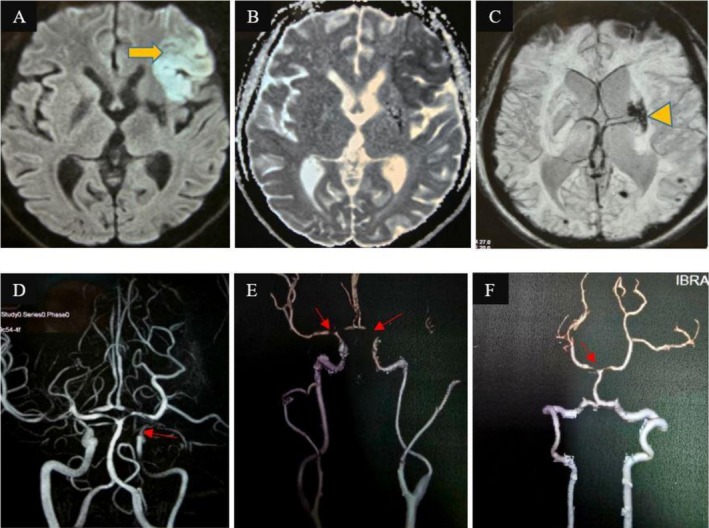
Follow up MRI of Brain, after 3 months demonstrated an area of restricted diffusion in left frontal lobe (A and B, marked by yellow arrow). Additionally, haemorrhagic transformation noted in left capsule‐ganglionic area (C, marked by yellow arrowhead). Both MRA (D) and CTA (E, F) demonstrated diffuse moderate to severe disease of left ICA, ipsilateral occlusion of M1/M2, A2 segment, right P1 and M1 segment (marked by red arrow). MRI, magnetic resonance imaging.
